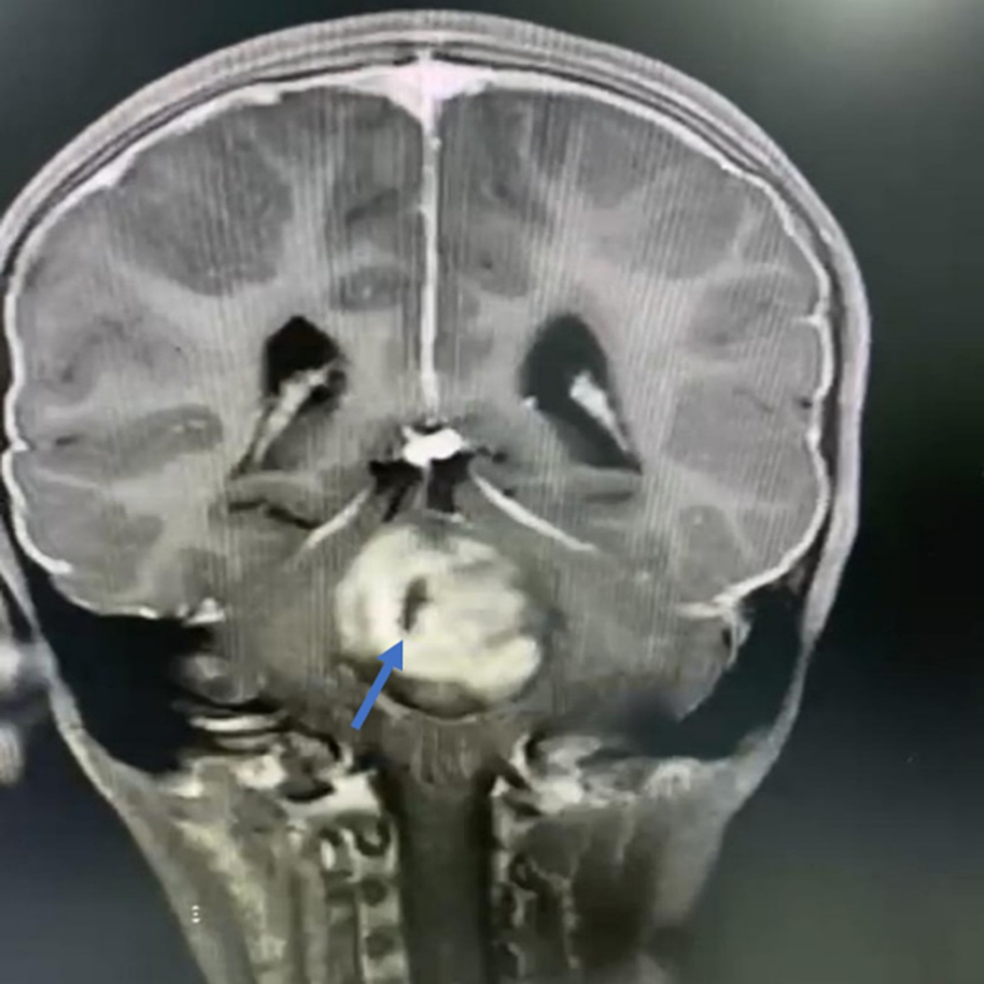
Preoperative image - MRI brain coronal cut shows obstructive hydrocephalus
